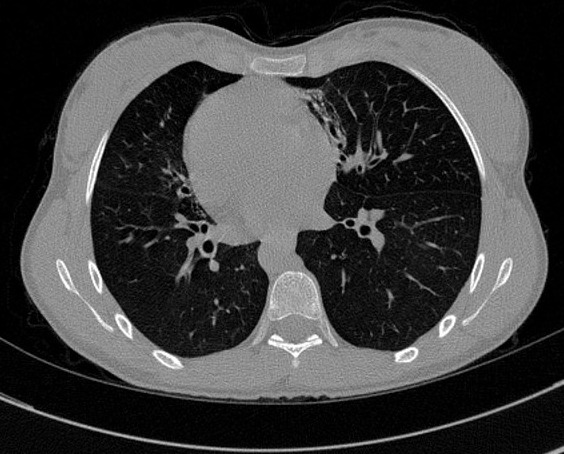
HRCT of the chest in parenchymal window and in axial section reveals bronchiectasis and dextrocardia
